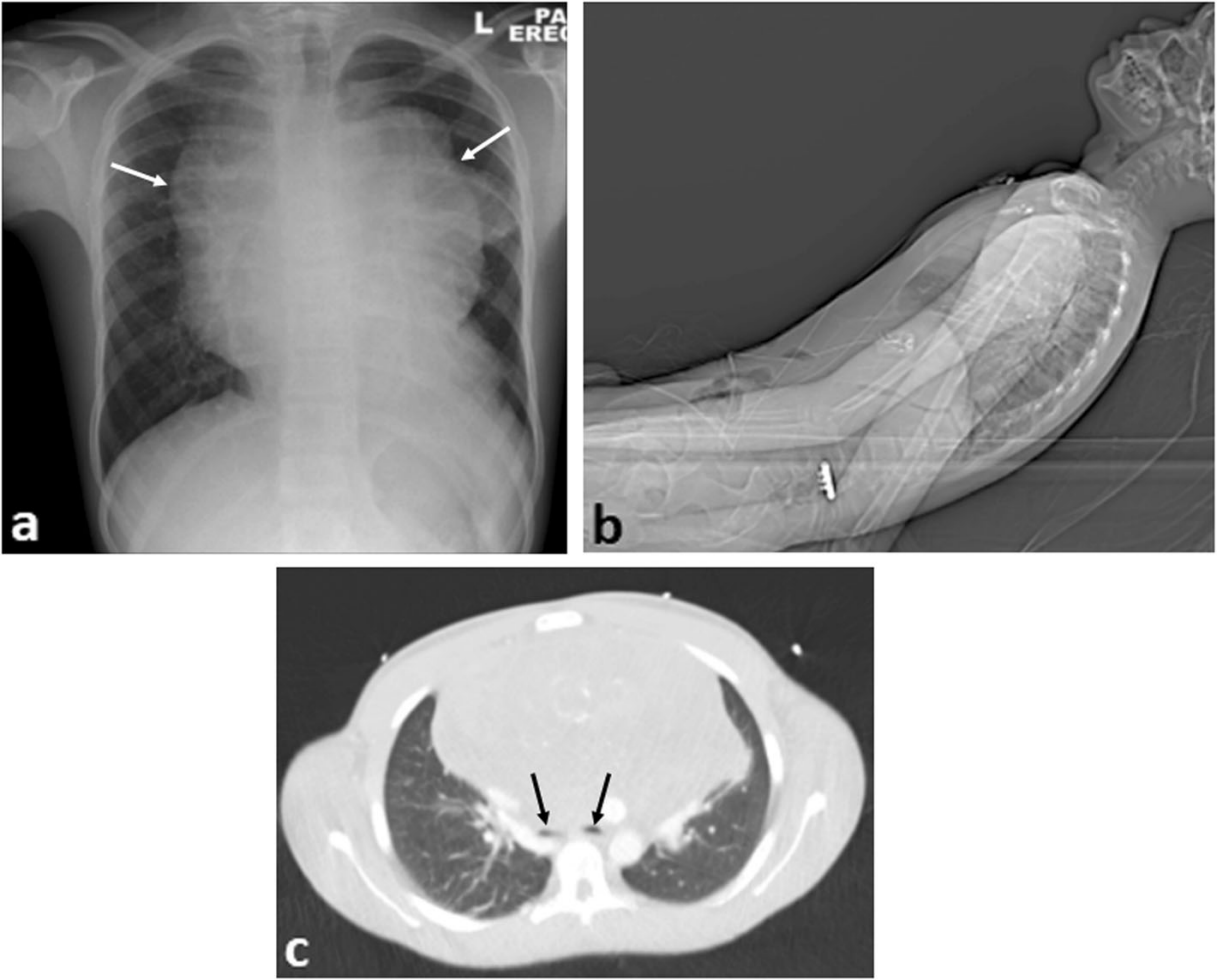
A 15-year-old boy with difficulty breathing and stridor presented with a large anterior mediastinal mass secondary to Hodgkin’s lymphoma. a Erect plain chest radiograph reveals a widened mediastinum (arrows). b Lateral view of the topogram from the CT scout image demonstrates the semi-upright positioning of the patient in the scanner because of reduced air entry on lying supine. c Axial CT imaging (lung windows) at the carina demonstrates marked airway compression of the main bronchi (black arrows) from the anterior mediastinal mass
